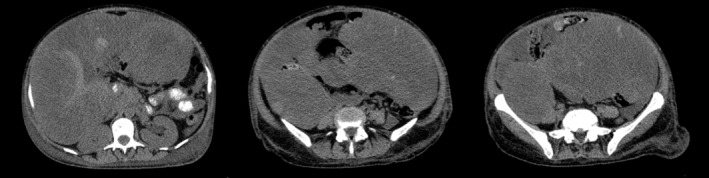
CT scan of abdomen and pelvic demonstrating multiple masses with extensive invasion to adjacent organs.
